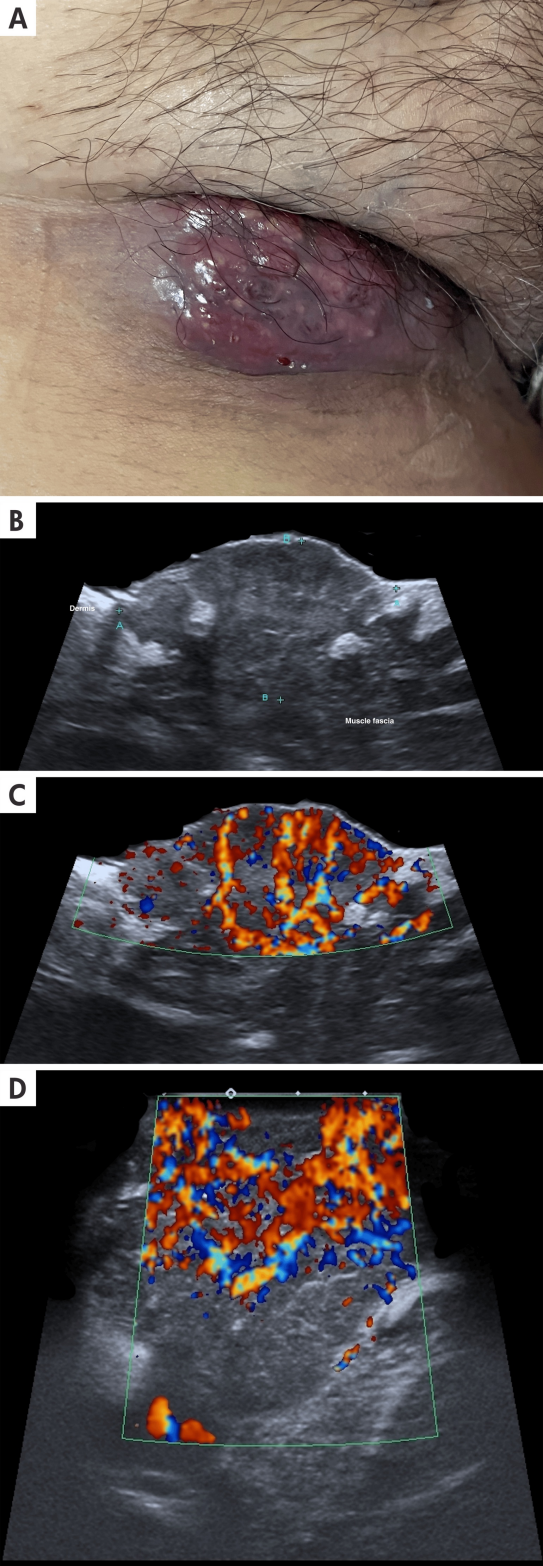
Dermatological ultrasound and clinical imaging of cutaneous angiosarcoma A: A 46-year-old female patient with a lobulated, edematous, red-violet, vascular-appearing tumor lesion, approximately 5 cm x 2 cm in size, with surrounding skin also violet and edematous, located in the right inguinal region. B: The longitudinal ultrasound view and grayscale ultrasound of the inguinal area show a mix of hypoechoic and hyperechoic structures with poorly defined borders. The calipers (A+) show the longitudinal extension, and the calipers (B+) show the anteroposterior borders. C and D: On Doppler, duplex color Doppler color ultrasound, there are multiple, tortuous vessels with irregular borders.
